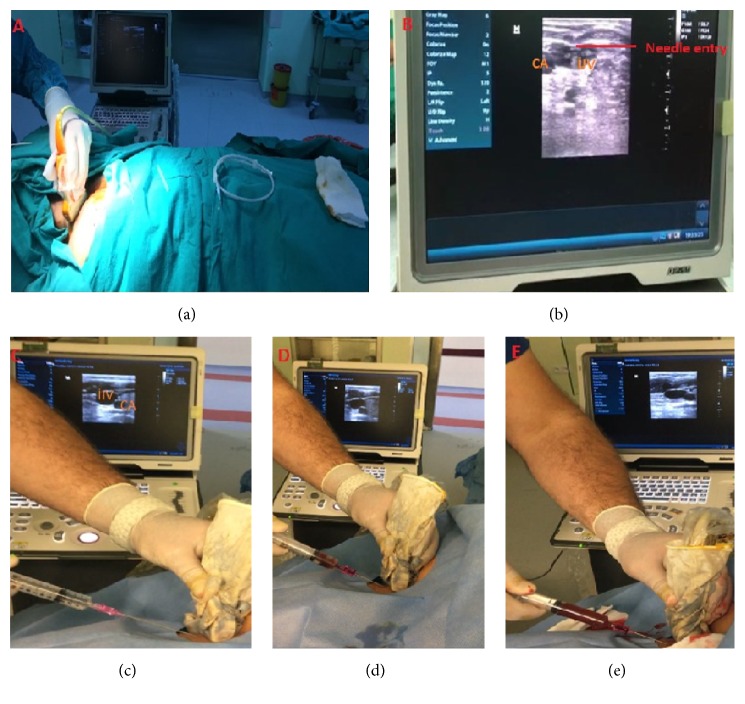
Venous intervention through USG method. Jugular vein is out of plane view. b, c, d, and e represent a needle that appears as a hyperechoic point on the ultrasound screen within the lumen of the jugular vein and entry appears in the blood of the injector.* IJV*, internal jugular vein;* CA*, carotid artery.
